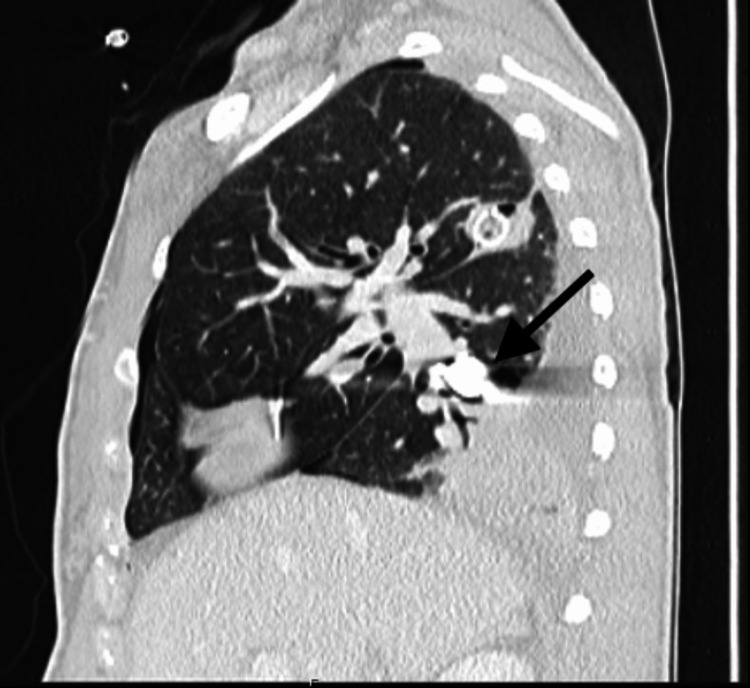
Sagittal CT chest reveals stable position of bullet with subsequent atelectasis in the posterior segment of the right lower lobe due to evolving infarction and collapse. Interval placement of the right apically oriented chest tube due to right hemopneumothorax.
